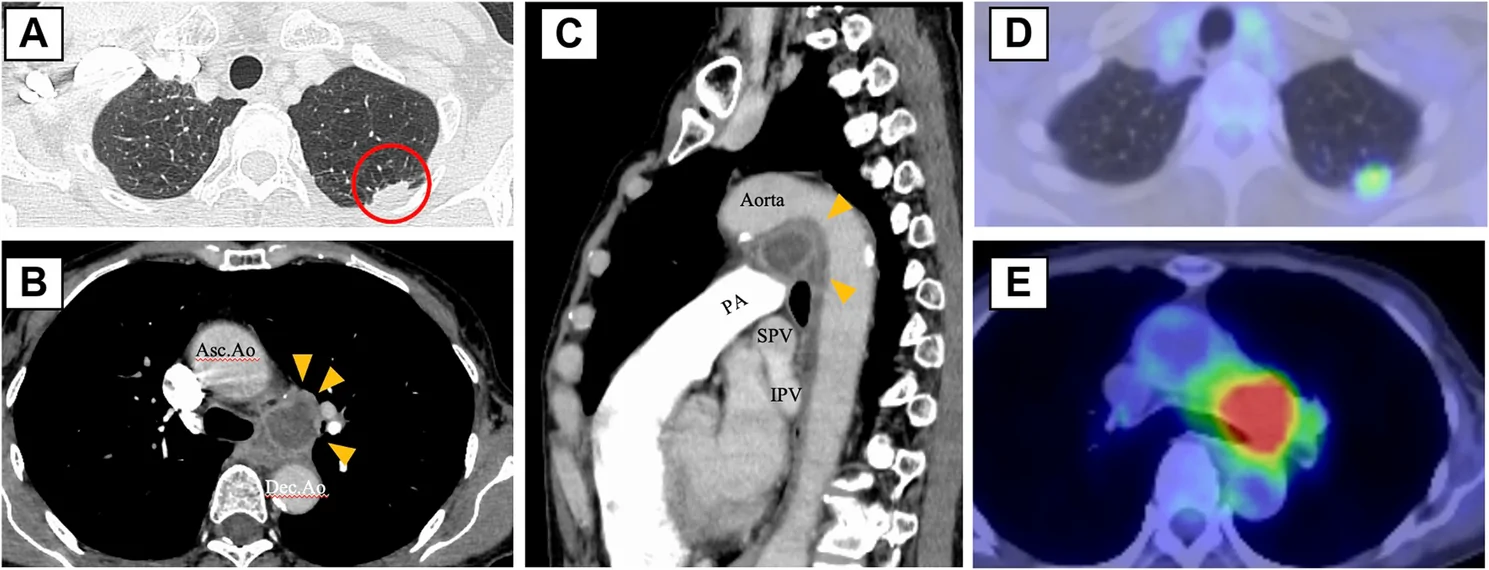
(A) Axial lung-window computed tomography (CT) demonstrates a 16-mm primary tumor in the left upper lobe (S1+2). (B) Axial and (C) sagittal mediastinal-window CT images reveal a bulky #5 lymph node abutting the aortic arch, with findings suspicious for direct invasion. (D, E) ¹⁸F-fluorodeoxyglucose (FDG) positron emission tomography (PET)/CT demonstrates intense FDG uptake in the primary tumor (D) and in the #5 lymph node (E), with no evidence of additional nodal involvement or distant metastasis
